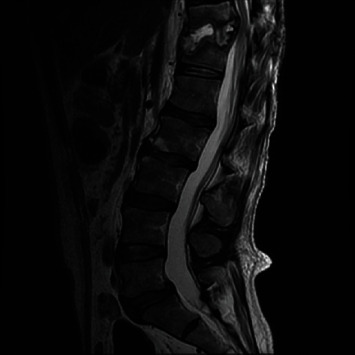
MR thoracic spine T2-weighted image 3 months later demonstrates progression of end plate destruction and phlegmon and progression of spinal stenosis.
